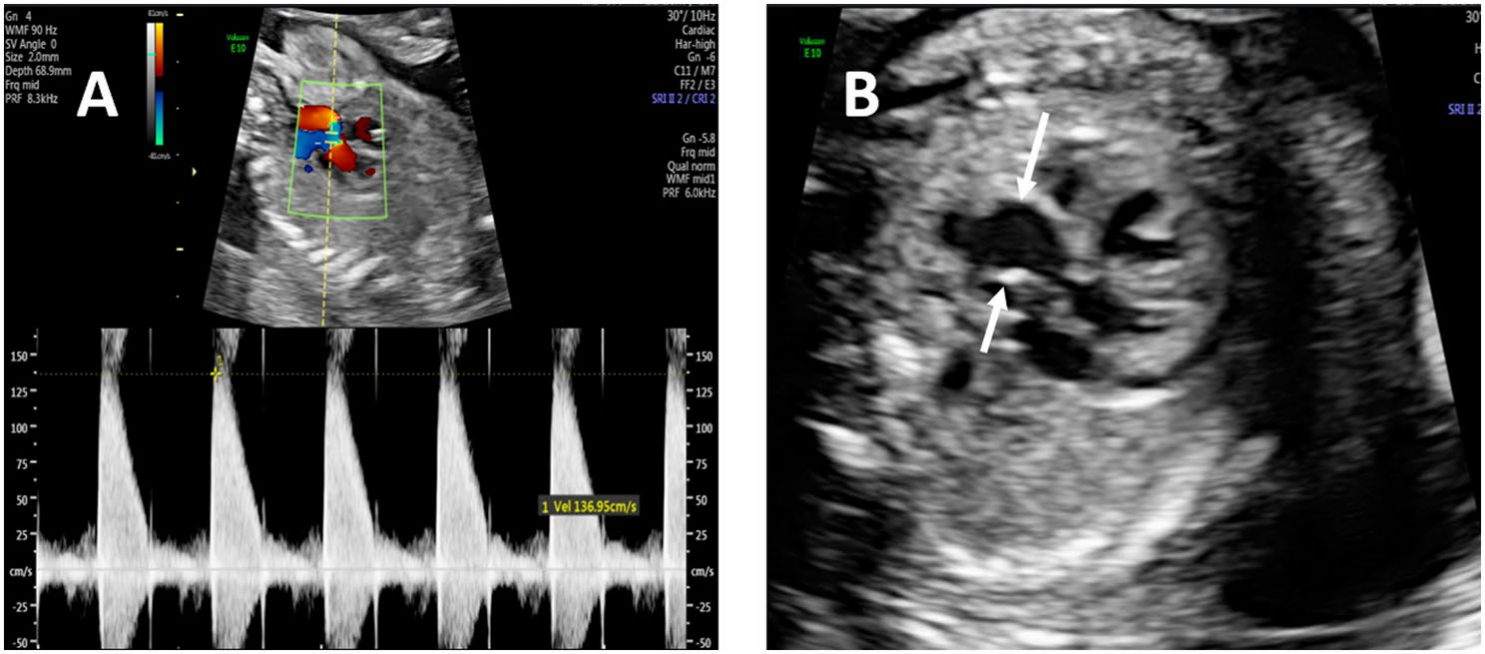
Indirect ultrasound signs of the fetal bicuspid aortic valve. Increased supra-aortic valve velocity (A). Post-stenotic widening of the ascending aorta (B)
